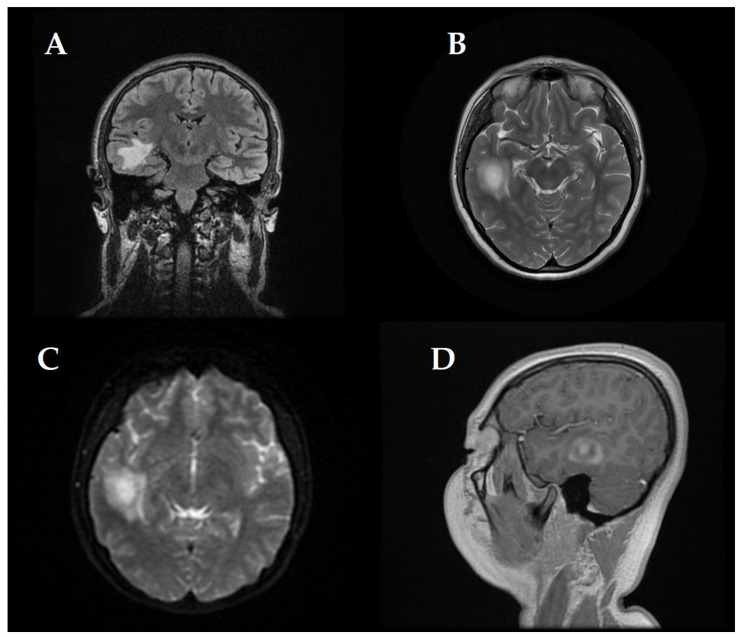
(A–D) First MRI shows (A) Coronal FLAIR sequence right temporal lesion, characterized by moderate peripheral vasogenic edema, Axial T2-weighted imaging (B) confirms the extent of edema around the lesion, (C) Diffusion-weighted imaging (DWI) reveals restricted water diffusion and Sagittal post-contrast T1-weighted imaging (D) highlights predominantly late peripheral contrast enhancement measuring 23 × 19 × 21.5 mm.
